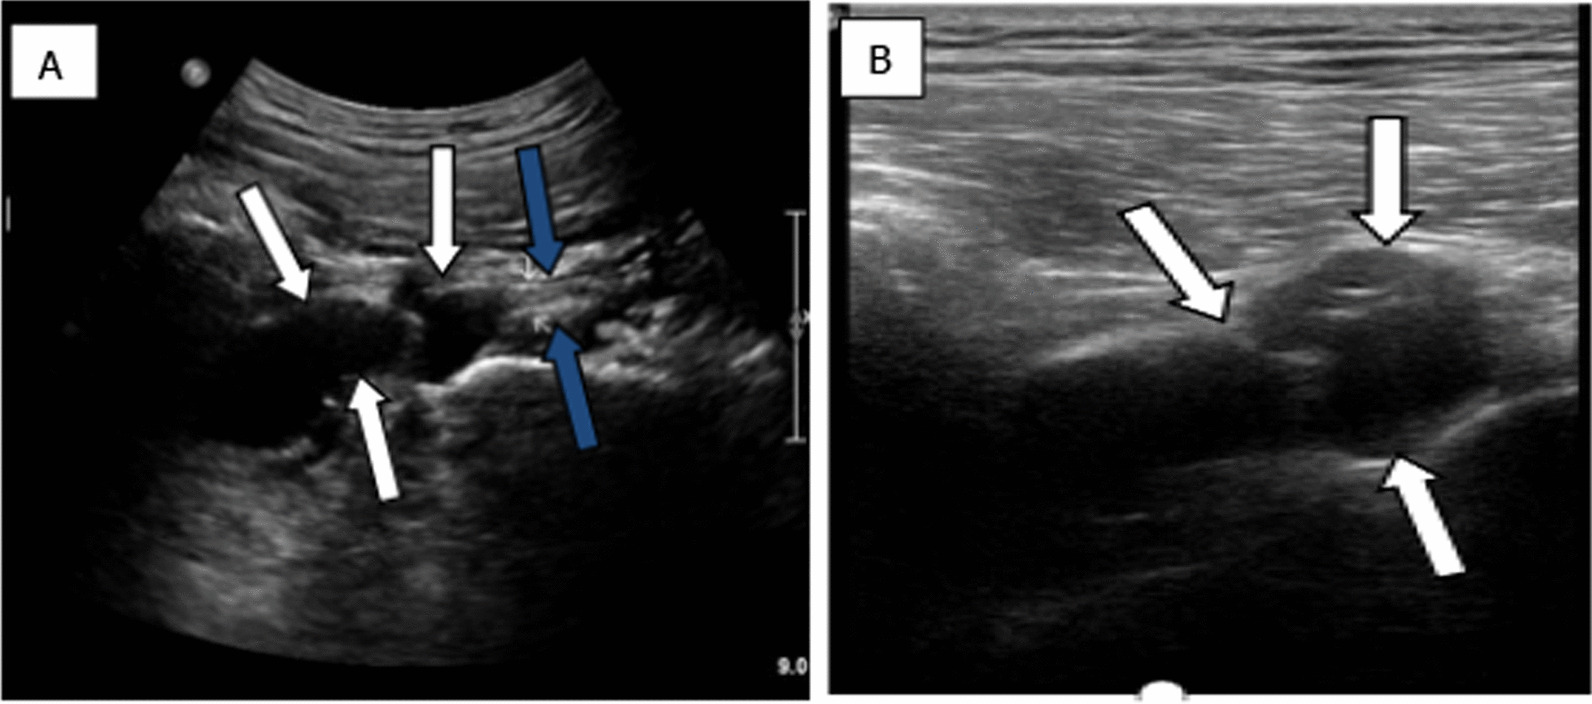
Sonographic examination showing a separate anechoic mass (white arrows) above the outlet of the right piriformis muscle pushing the right sciatic nerve (blue arrows). A Sagittal view(low- frequency probe); B Transverse view (high-frequency probe)
